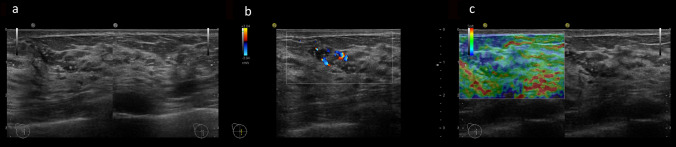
A 46-year-old woman with a *BRCA2* mutation and ductal carcinoma in situ (luminal-HER2 subtype). Breast ultrasound demonstrates a hypoechoic area with calcifications. We defined this morphology as no mass. The hypoechoic area shows high vascularity and score 5 elasticity (no strain in the entire lesion or surrounding area). *HER2* human epidermal growth factor receptor 2
